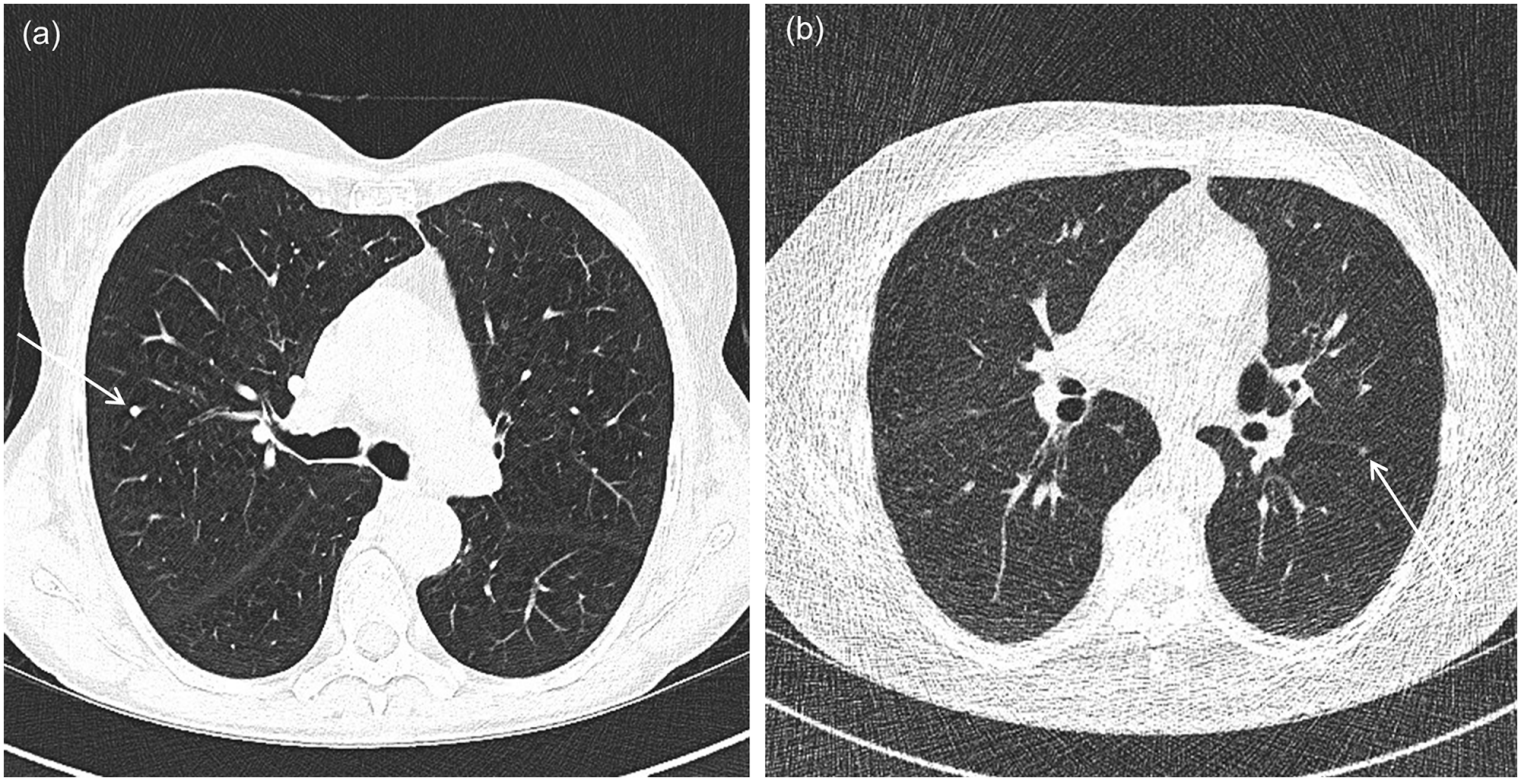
Representative transverse CT sections of a female patient with a nodule (arrow) scanned with a standard dose protocol (lung dose 5.6 mGy) (a), and a male patient with a ground glass nodule at the fissure (arrow) scanned with a low-dose CT protocol (lung dose 0.44 mGy) (b).
